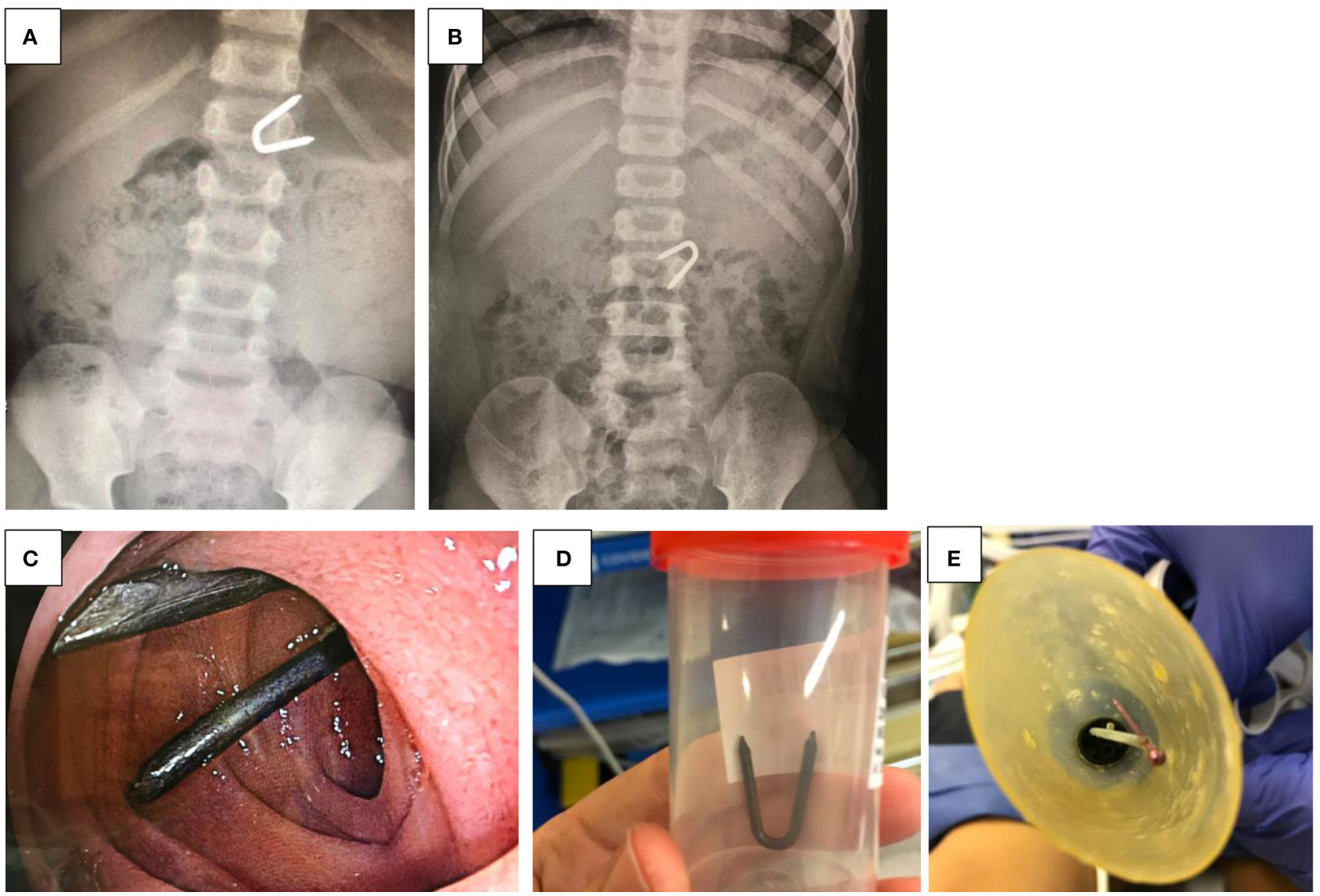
(A–E) Ingestion of a pointed foreign body. (A,B). Abdominal x-ray of a pointed foreign body ingested by a 5-year old child. (C) Foreign body in the mid-duodenum. (D) FB after successful extraction with a retrieval forceps. (E) Foreign body (pin to fasten clothing) which has been grasped *via* a polypectomy snare and withdrawn into a protector hood.
